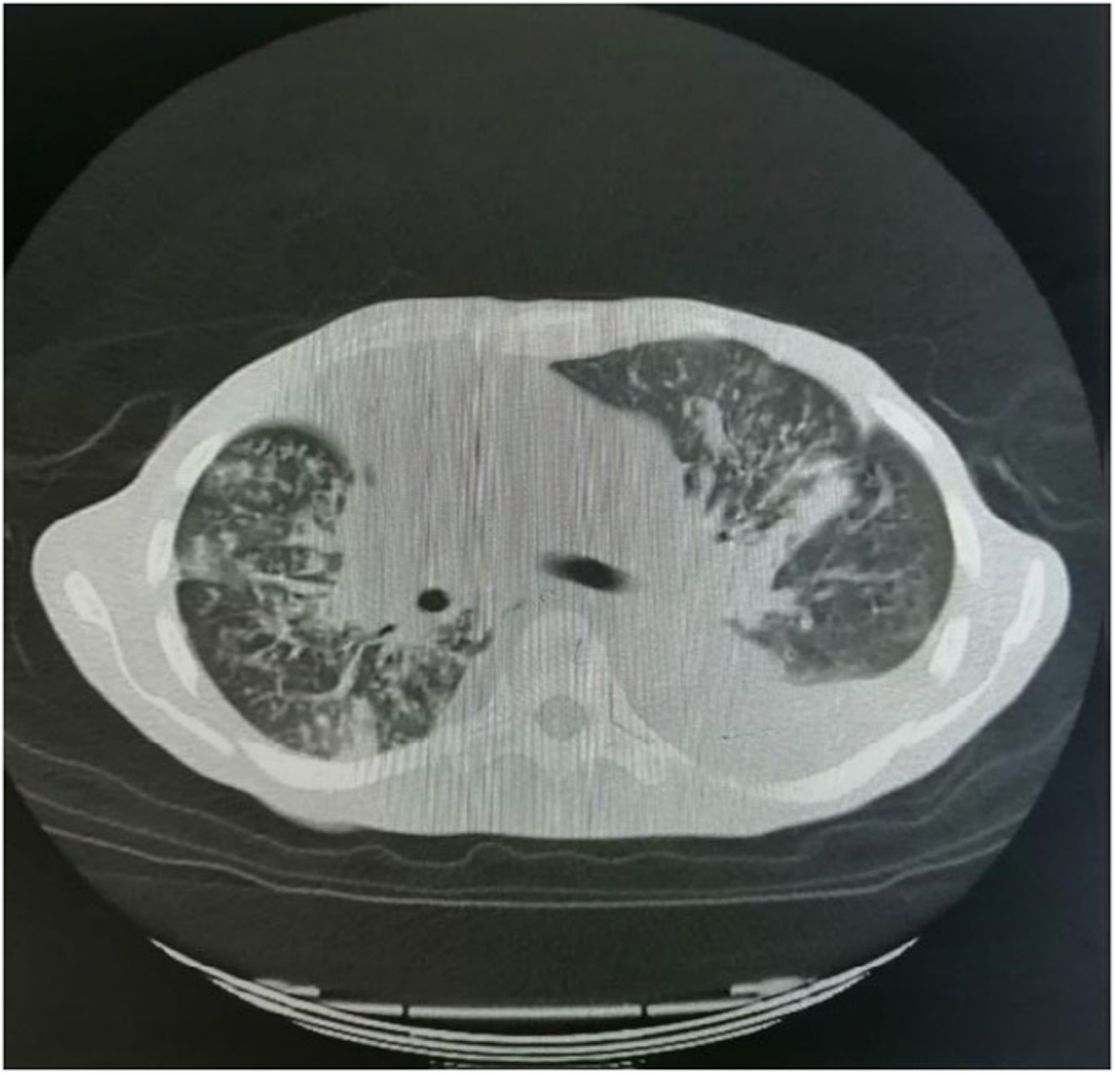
High resolution computed tomography (HRCT).
